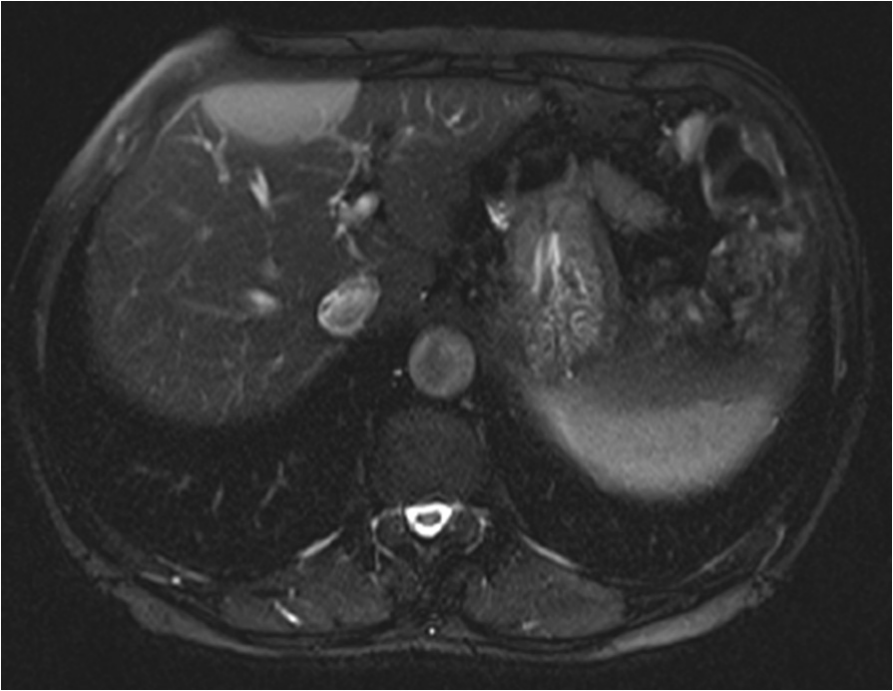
Axial T2-weighted with fat suppression magnetic resonance imaging (MRI) scan showing a mass that demonstrates moderate homogenous hyperintensity, but that is iso-intense when compared with spleen parenchyma.
